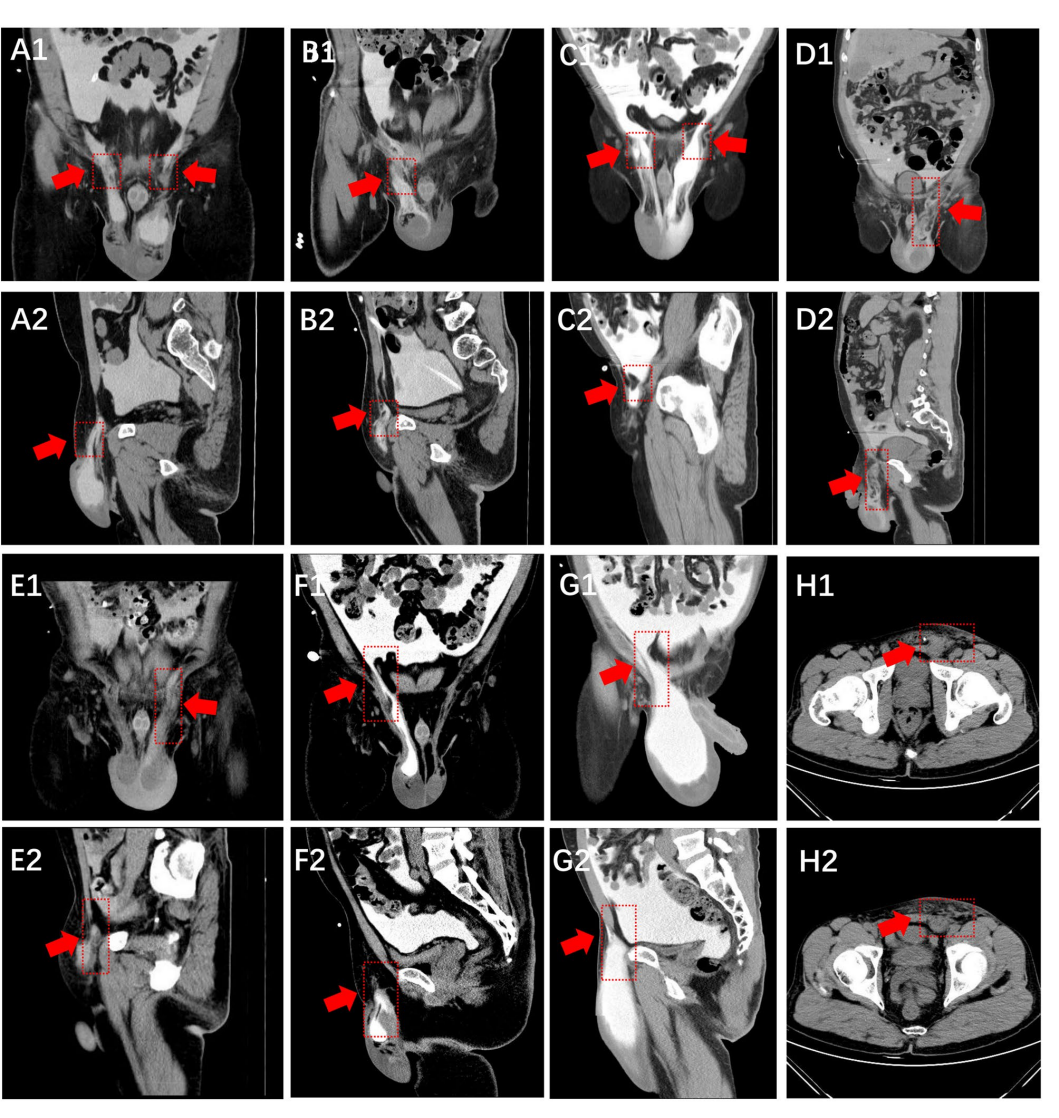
Summary of Imaging Findings from CT Peritoneography for Diagnosis and Leakage Pathways in 8 Cases of Scrotal Leak. Label 1 indicates imaging results in the coronal plane. Label 2 indicates imaging results in the sagittal plane. (Labels H1 and H2 are unique, representing imaging results in the axial plane for Case 8. This was our first patient undergoing CT peritoneography. The image quality is inferior to the subsequent seven cases, likely because our early inexperience led to initiating the CT scan immediately 30 min after contrast agent injection. For the later 7 patients, the contrast agent was allowed to remain in the peritoneal cavity for 2–3 h, which significantly improved imaging quality.). Labels A through H correspond to Case 1 through Case 8, respectively. For detailed radiological descriptions and all corresponding images, please refer to Supplement Appendix 1
